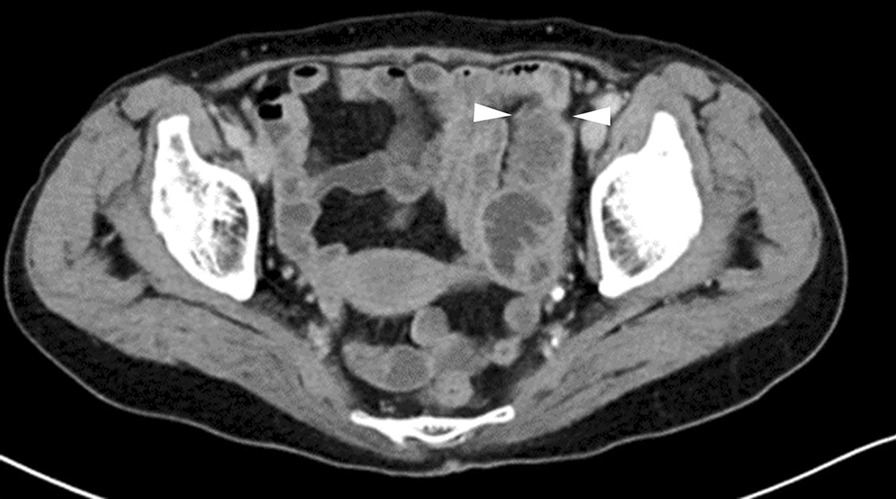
Abdominal computed tomography (CT) showed slight thickening of the small intestinal wall (arrow head)
